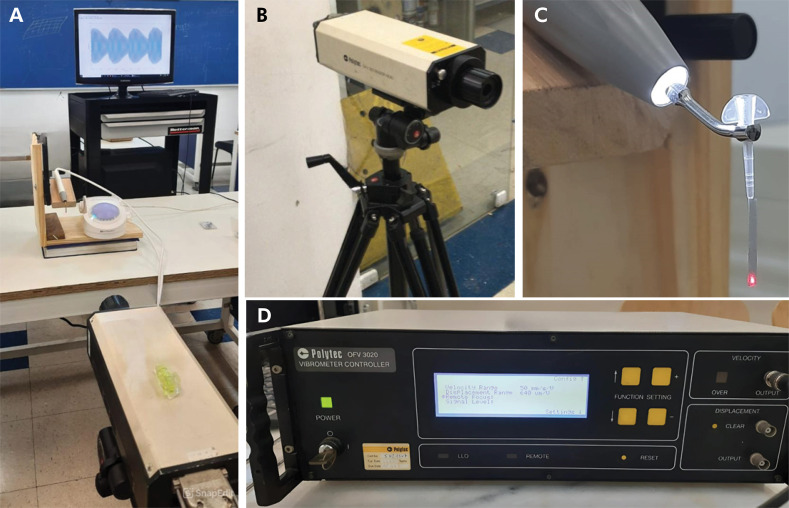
A.: Execution of the experiment, using the laser Doppler vibrometer. B.: Laser model OFV-323. C.: Handpiece positioned on the support for data acquisition. D.: Vibrometer controller model OFV-3020.
